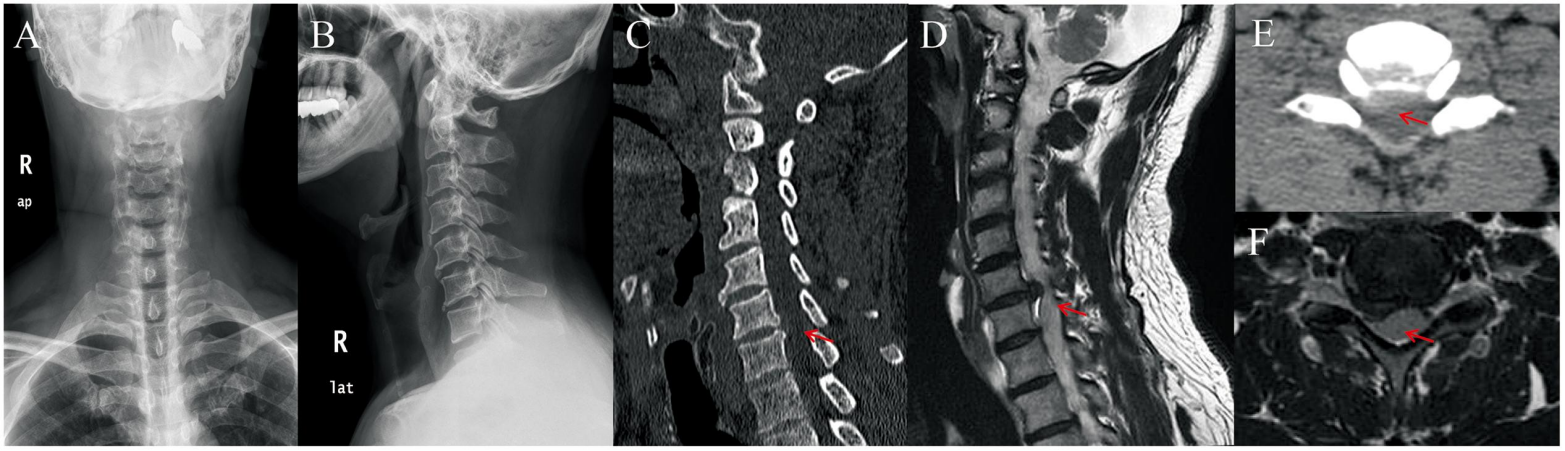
Preoperative imaging of the C6/C7 disc herniation. (A) Anteroposterior radiograph showing preserved cervical alignment without scoliosis or deformity; (B) lateral radiograph demonstrating normal cervical lordosis and absence of segmental instability; (C) sagittal CT reconstruction revealing disc space narrowing and right paracentral osteophyte formation at the C6/C7 level (red arrow); (D) sagittal T2-weighted MRI showing a right paracentral C6/C7 disc herniation compressing the exiting C7 nerve root (red arrow); (E) axial CT image confirming right-sided disc-osteophyte complex encroaching on the lateral recess (red arrow); (F) axial T2-weighted MRI demonstrating focal right C6/C7 disc protrusion compressing the C7 nerve root (red arrow).
